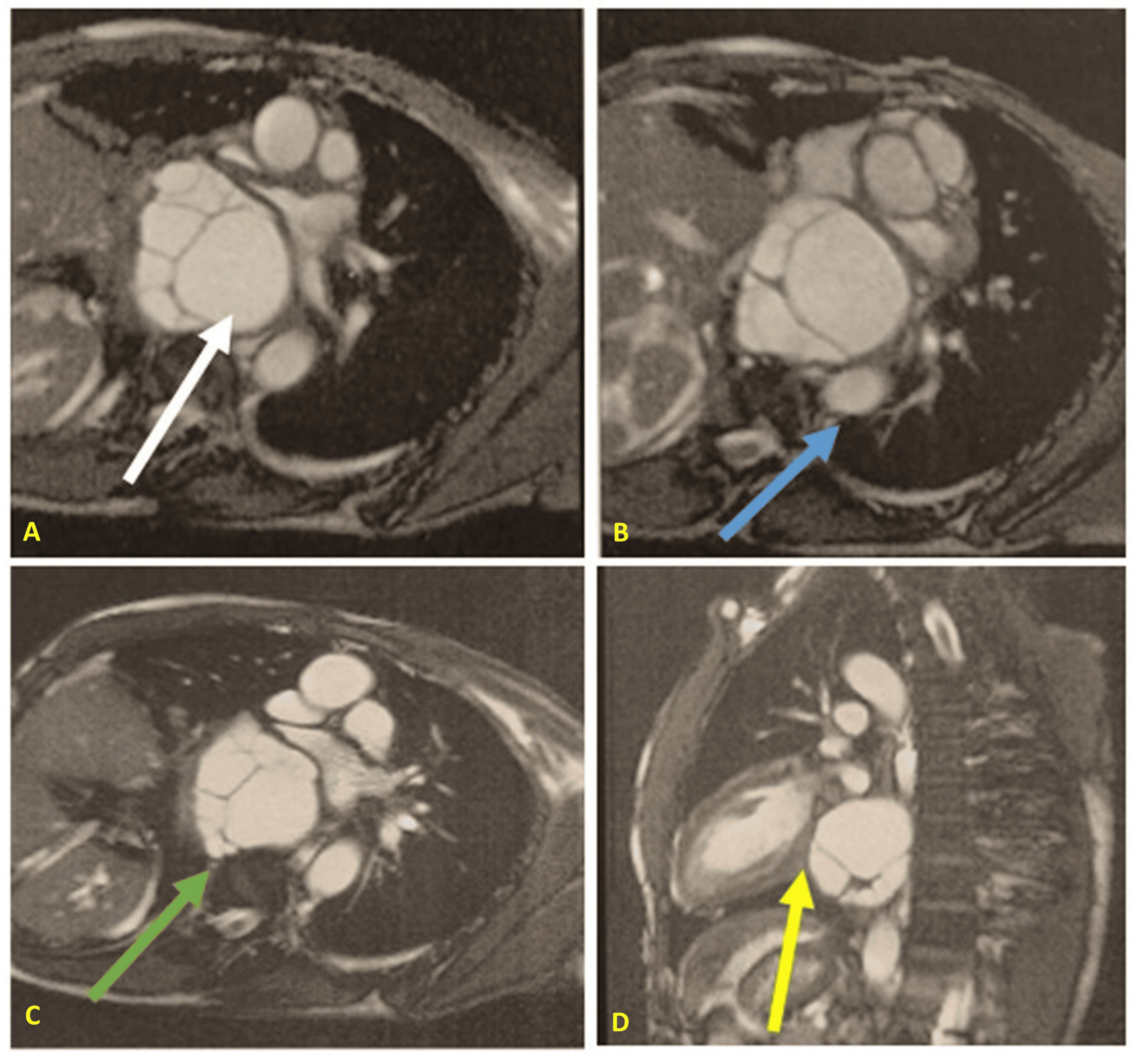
Magnetic resonance imaging shows a cyst located in the posterior-inferior mediastinum (Image A, white arrow), in close contact with the spine but without evidence of spinal cord invasion (Image C, green arrow). The cyst compresses the atria anteriorly without signs of infiltration (Image D, yellow arrow) and displaces the aorta to the left (Image B, blue arrow).
